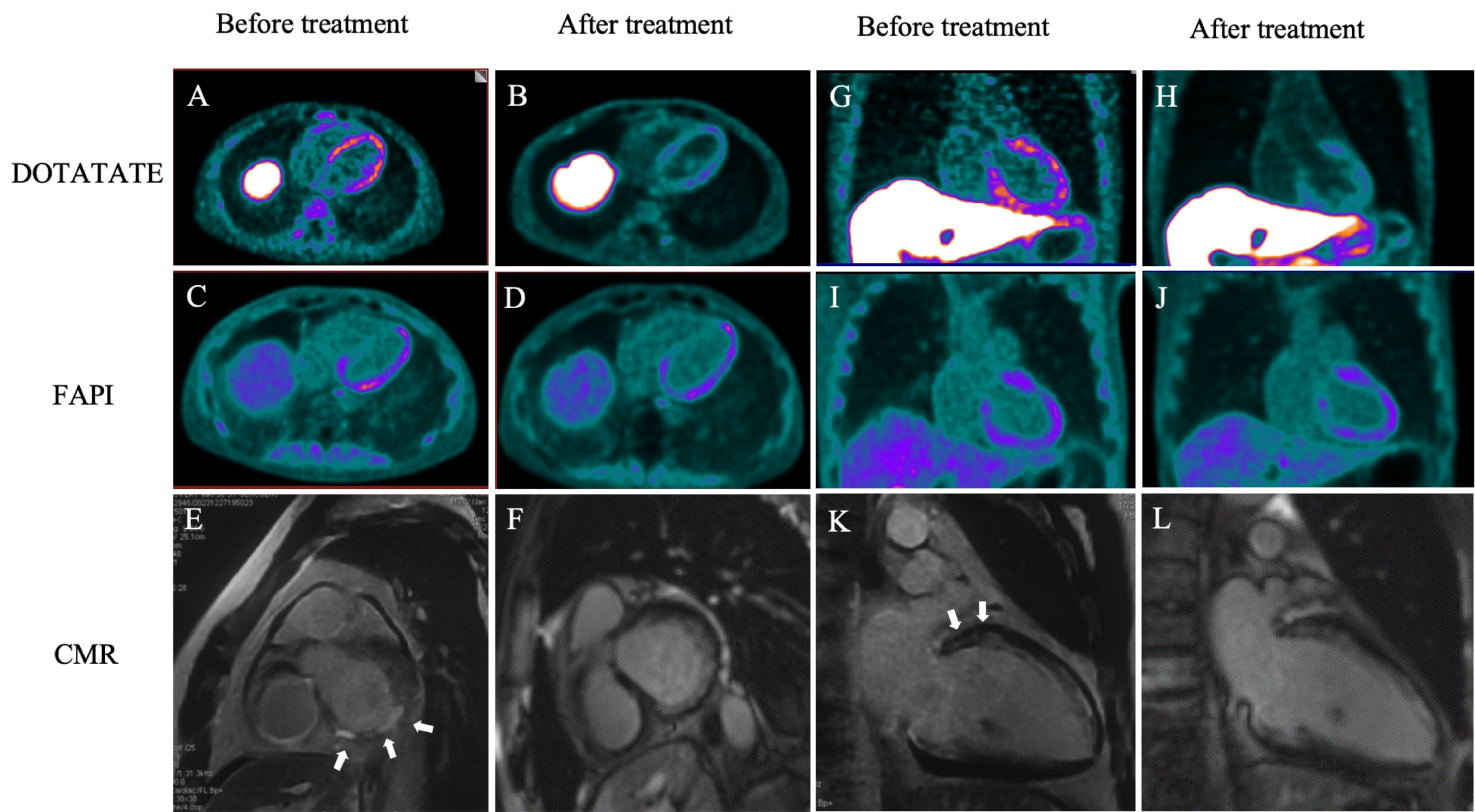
Longitudinal imaging assessment of myocardial inflammation and fibrosis before and after immunosuppressive therapy. (A, B, G, H) ^68^Ga-DOTATATE PET/CT images. Baseline imaging (A, G) demonstrates diffuse myocardial uptake in the interventricular septum and left ventricular wall (SUVmax 4.04), indicating macrophage-driven inflammation. Post-treatment (B, H), this uptake is significantly reduced (SUVmax 2.51), consistent with resolved inflammation. (C, D, I, J) Al18F-NOTA-FAPI-04 PET/CT images. Baseline (C, I) shows focal, heterogeneous uptake in the anterior, lateral, and inferior walls (SUVmax 3.79), suggestive of fibroblast activation. Post-treatment (D, J), the focal uptake persists with a slight increase in SUVmax (4.18), indicating residual fibrotic burden. (E, F, K, L) Cardiac magnetic resonance (CMR) with late gadolinium enhancement (LGE). Baseline short-axis (E) and two-chamber long-axis (K) views show patchy mid-wall LGE (arrows), indicative of active inflammation or fibrosis. Follow-up CMR (F, L) after therapy shows partial resolution of the LGE (arrows).
